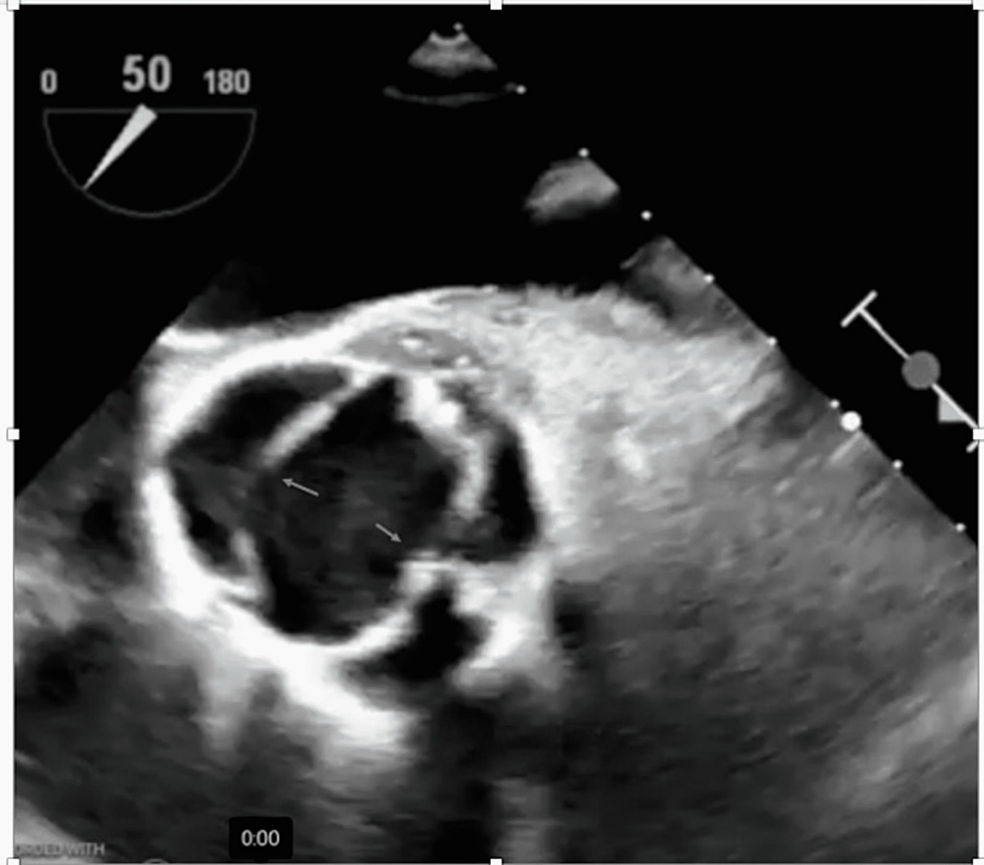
Transesophageal echo showing aortic valve with two leaflets (arrows) in the open position confirming bicuspid aortic valve in the short-axis view
